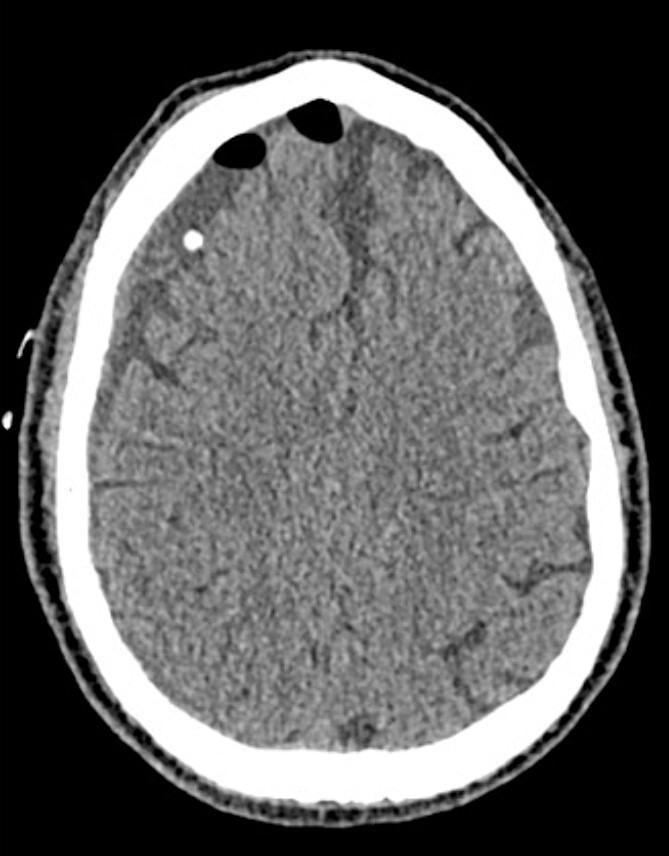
Postoperative CT imaging from Case 2.
